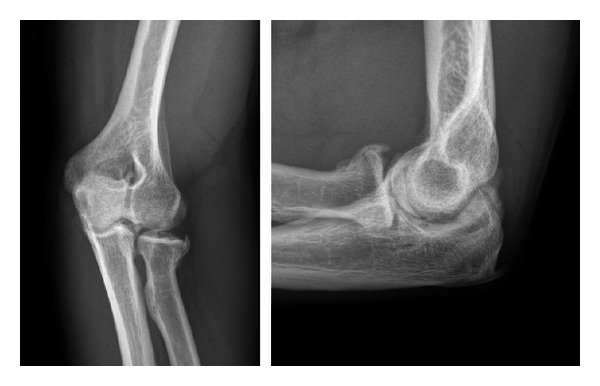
Oblique and lateral radiographs of a patient who sustained a radial head fracture treated nonoperatively; this patient later developed lateral elbow pain with forearm rotation. Radiographs demonstrate anterolateral osteophytes noted at the radial head and decreased joint space in the radiocapitellar joint.
